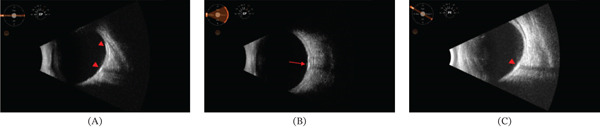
B‐scan ocular ultrasound of the left eye at the first visit. (A–C) Ocular wall calcification (red arrows) is shown with posterior acoustic shadowing involving the left macular region.
